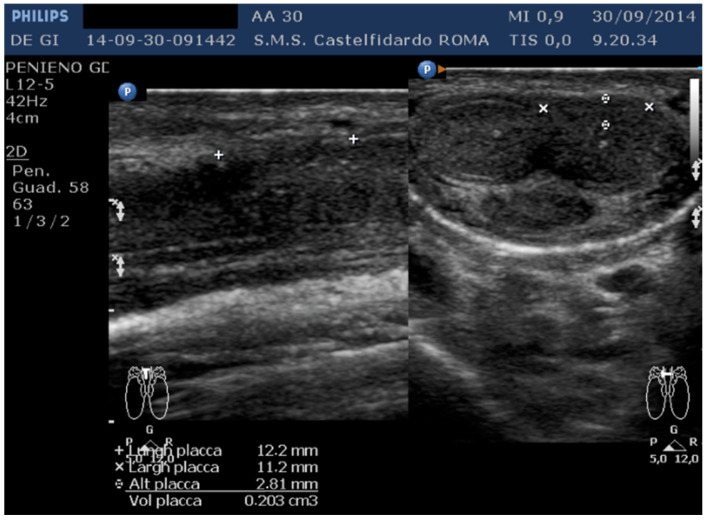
Ultrasonography of the penis and measurement of plaque before treatment (longitudinal and transverse views).
